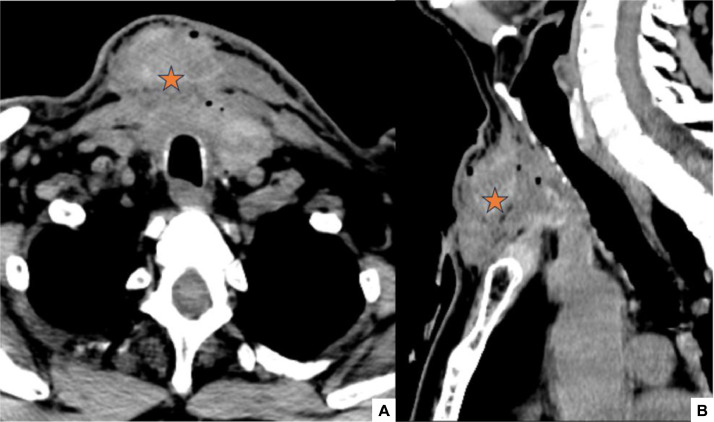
Axial (A) and Sagittal (B) pre-contrast cervical CT images on admission : Large and heterogenous hematoma in the anterior neck space, containing air bubbles (asterisk).
